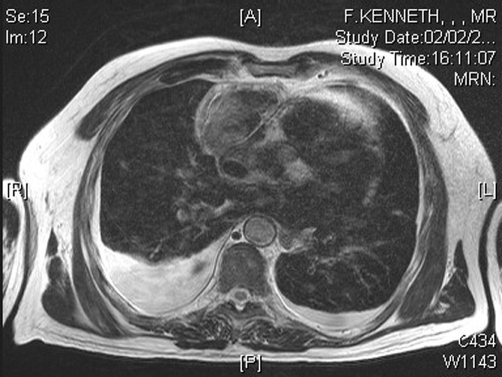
MRI scan showing the anterior mediastinal mass with associated haemorrhage and bilateral pleural effusions.
